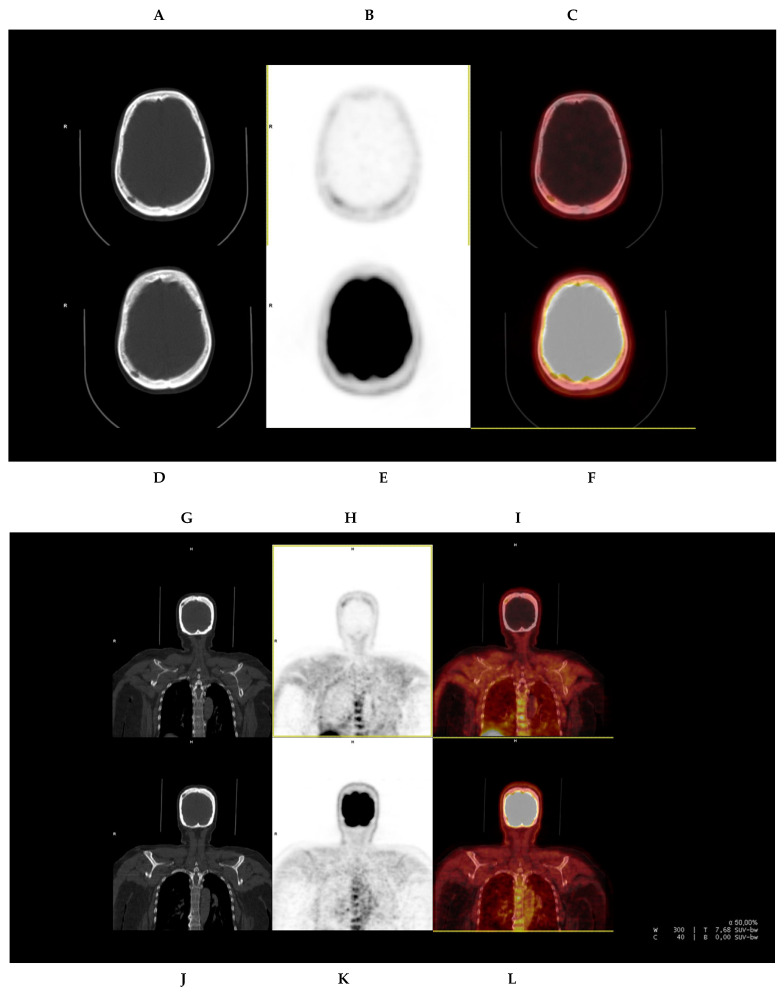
Fifty-nine year-old male (patient #7) presenting multiple lytic lesions located in the cranial calvarium. [^18^F]FCH PET/CT (upper row, (A–C,G–I)) and [^18^F]FDG PET/CT (lower row, (D–F,J–L)) are presented, corresponding to the axial (A–F) and coronal (G–L) projections of CT, PET, and PET/CT fusion, respectively. The images show [^18^F]FCH uptake in the lytic lesions (SUVmax 1.6), which had no [^18^F]FDG uptake. These differences are evident in both the axial and coronal projections. The [^18^F]FDG PET/CT and [^18^F]FCH PET/CT images are presented with equal grey-scale intensity.
